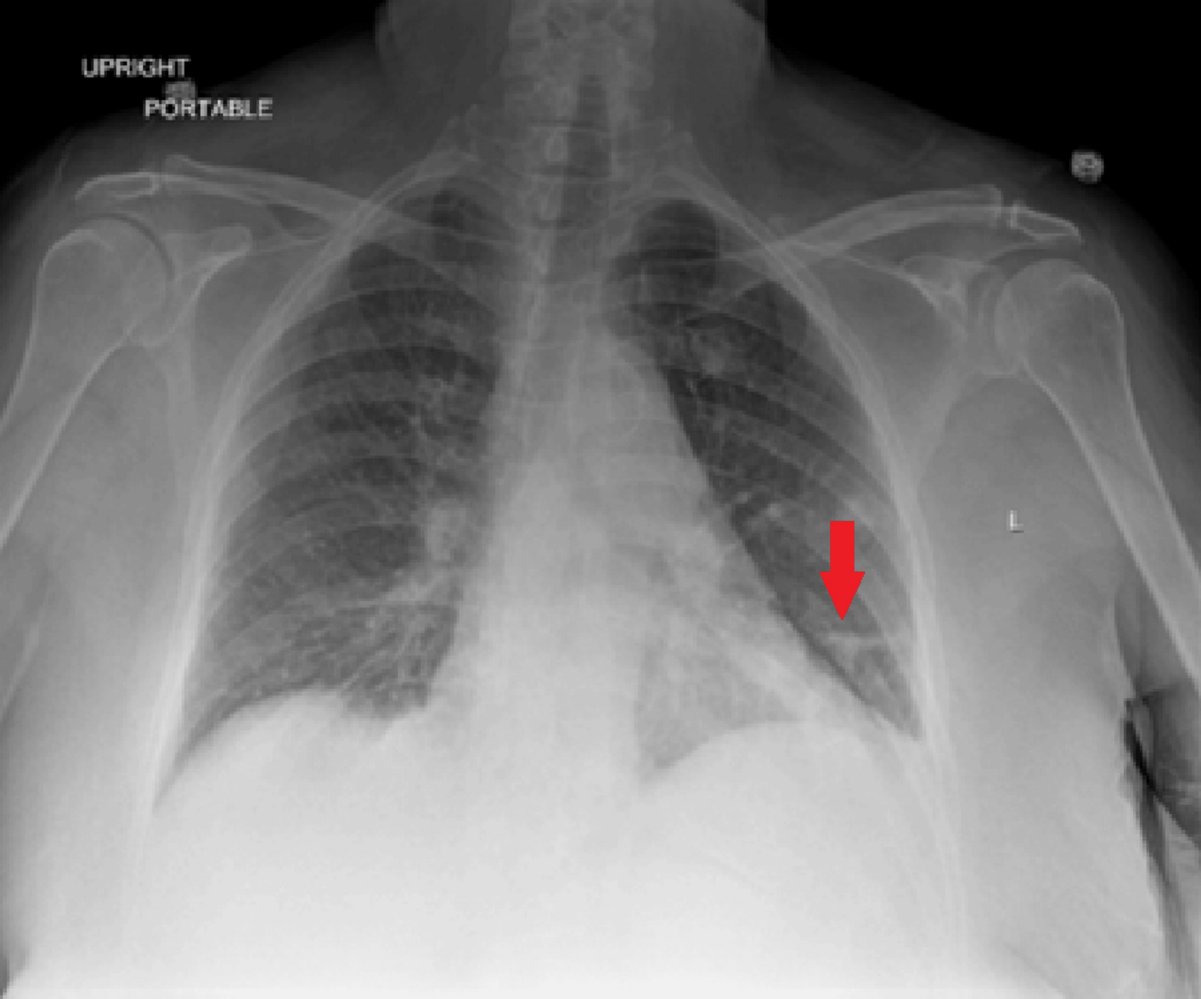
Chest X-ray showing worsening of the left lower lobe infiltrate (shown by red arrow).
